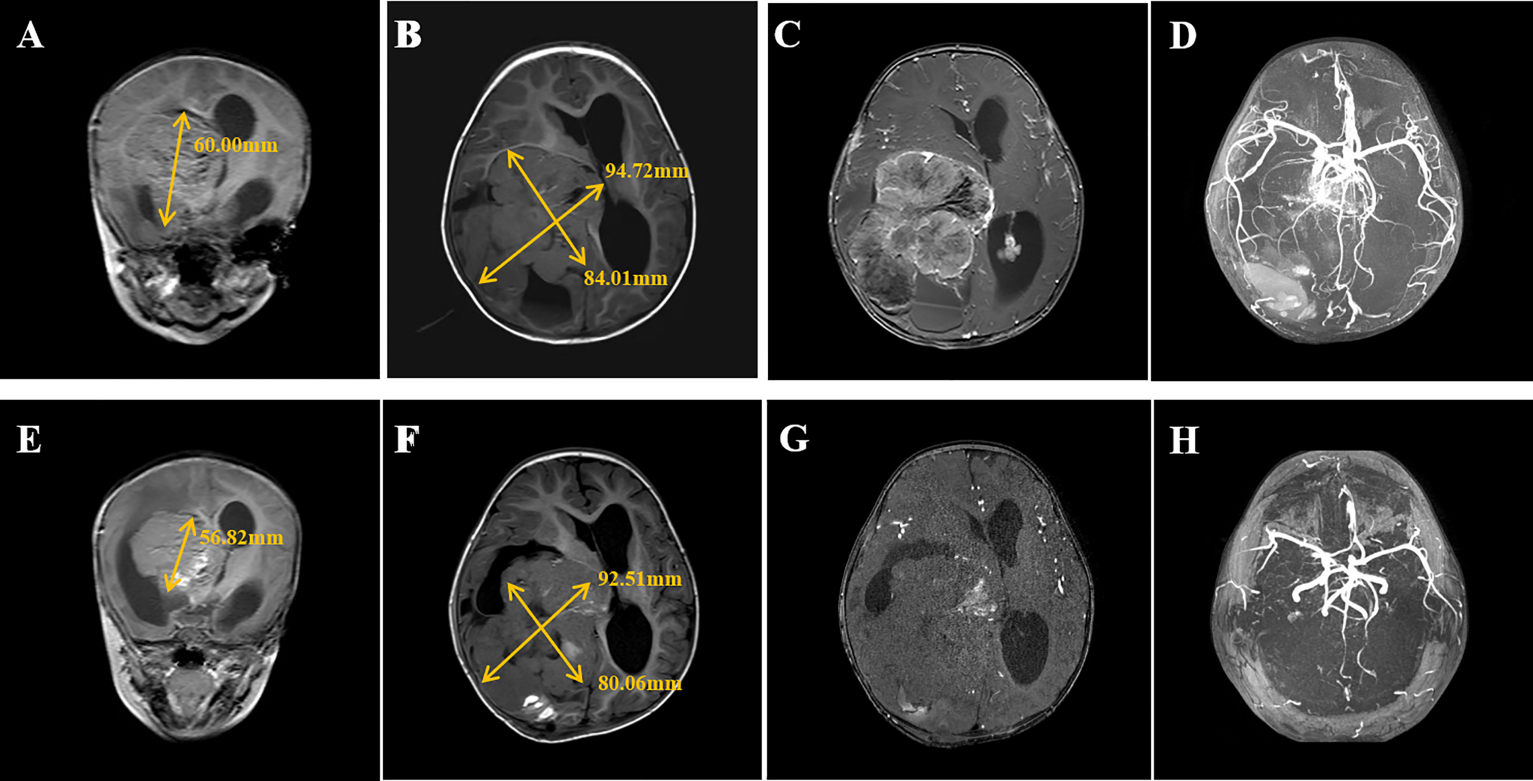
Serial intracranial MR images of the patient. Panel 1 Coronal (A) and transverse (B) T2-weighted MRI of the patient showed the tumor size in the right lateral ventricle and (C, D) MRA displayed the tumor with abundant blood supply (high-signal intensity) at admission (May 4, 2021). Panel 2 Coronal (E) and transverse (F) T2-weighted MRI showed a slight decrease in tumor size and (G, H) MRA displayed significant decrease of blood supply (high-signal intensity) to tumor after 14 days of oral rapamycin treatment (May 21, 2021).
